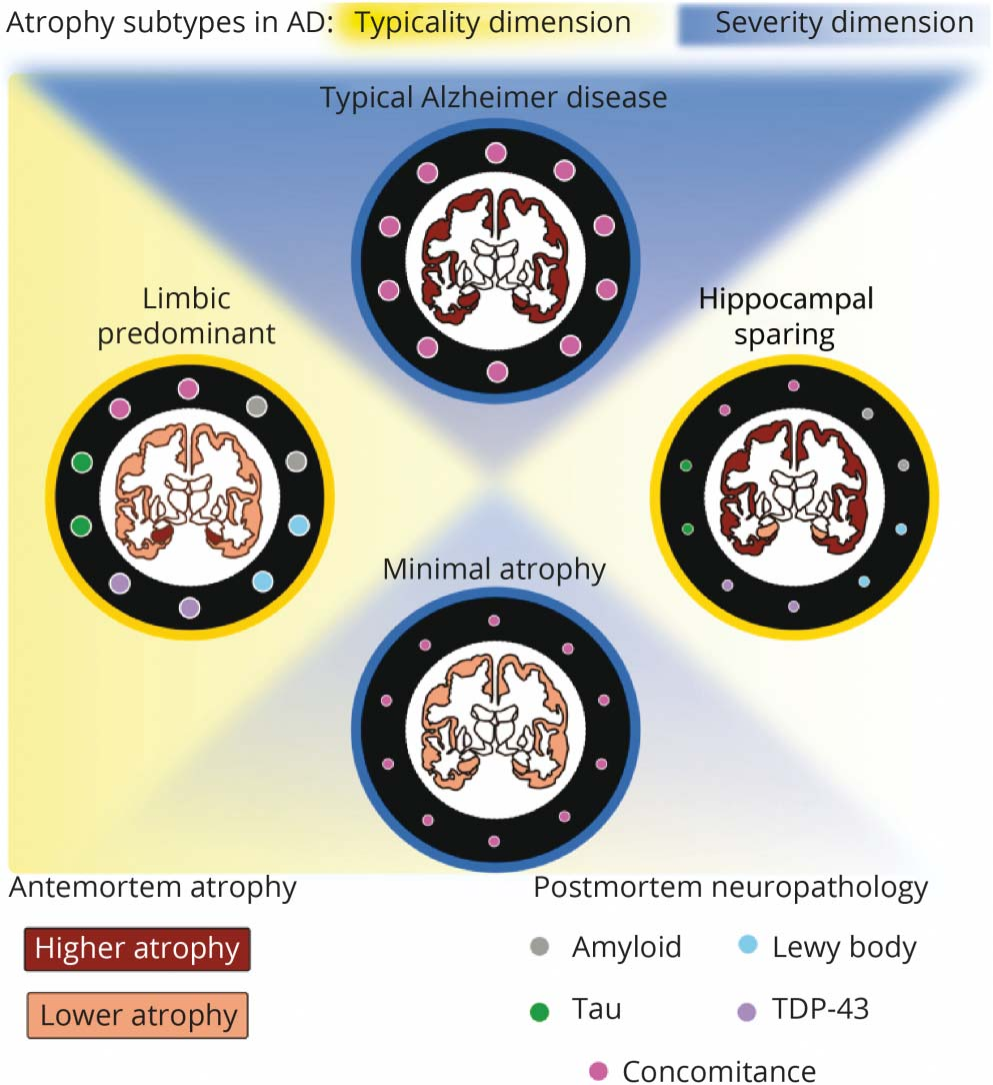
Susceptibility of Antemortem MRI-Based Heterogeneity to AD and Non-AD Neuropathologies Associations of antemortem typicality and severity with postmortem neuropathologic features may generate the following hypotheses: (1) the orthogonal dimensions of biological heterogeneity, typicality and severity, may offer complementary information regarding the vulnerability of the brain to AD (amyloid, tau) and non-AD (α-syn, TDP-43) pathologies; and (2) limbic-predominant AD along the typicality dimension and typical AD along the severity dimension may share similar underlying biological pathway(s), which make them more susceptible to pathologies, whereas hippocampal-sparing AD along the typicality dimension and minimal AD along the severity dimension may share similar pathway(s), making them less susceptible. α-syn = α-synuclein; AD = Alzheimer disease; TDP-43 = TAR DNA-binding protein 43.
